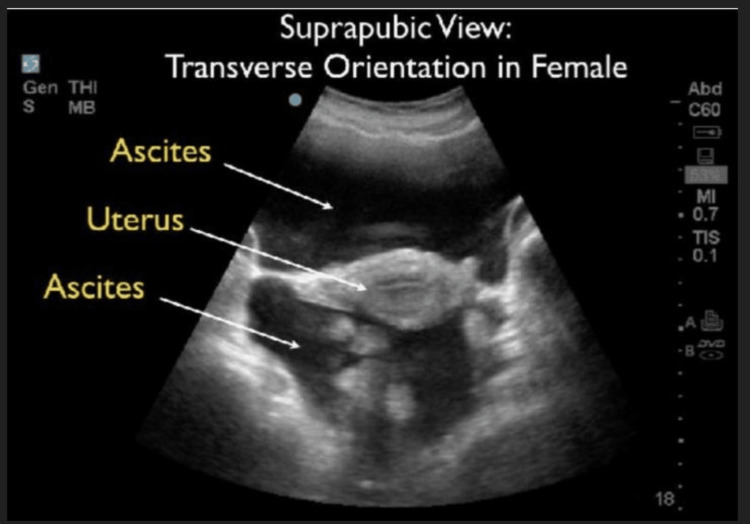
Transverse suprapubic ultrasound view revealing free fluid (ascites) above and below the uterus.
